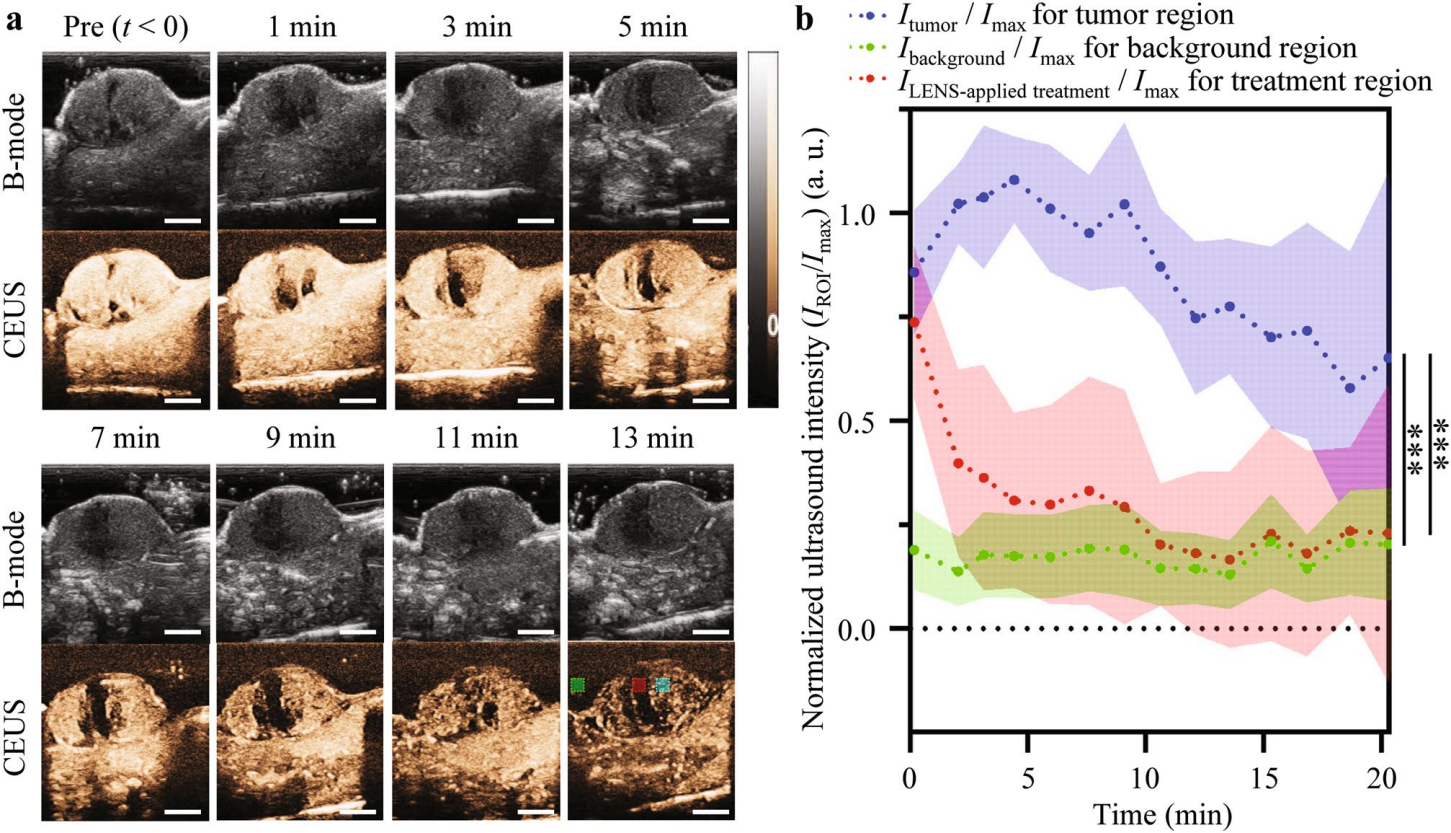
Contrast-Enhanced Ultrasound Imaging of Subcutaneous Tumors under FUS Exposure. (a) Time-dependent in vivo representative subcutaneous tumor US images after intravenous injection of the contrast agent (MB) under FUS exposure (Upper panel: B-mode; lower panel: CEUS-mode) (n = 1). Green, red, and cyan dotted boxes in the CEUS image at 13 min indicate region of interests (ROIs) of background, LENS-applied treatment, and tumor areas for the normalized US intensity calculation. (b) The normalized US intensity value of CEUS imaging, which is obtained by dividing US intensity in the ROI by the maximum US intensity in the entire CEUS image after injection of the contrast agent over time (*I*_tumor_/*I*_max_ vs. *I*_background_/*I*_max_: p* < 0.0001; *I*_tumor_/*I*_max_ vs. *I*_LENS-applied treatment_/*I*_max_: p* < 0.0001). The spot and shaded area represent the mean and standard deviation of the calculated values.
